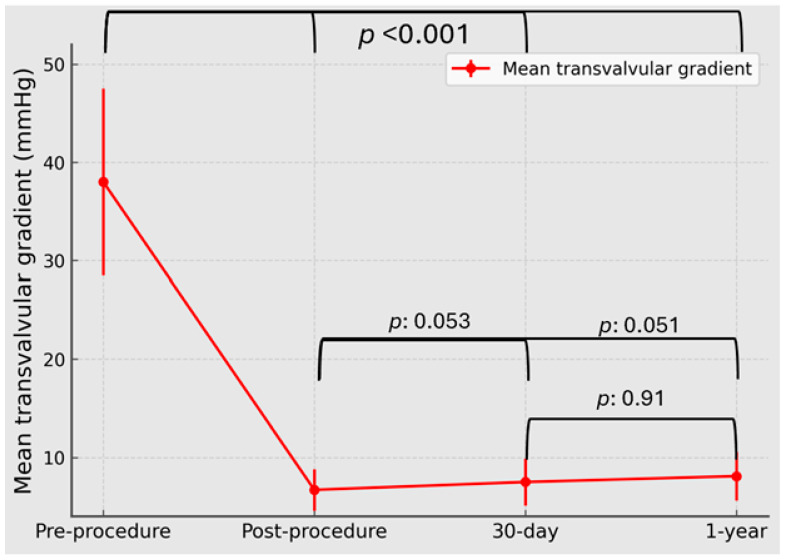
Serial mean transvalvular gradient after MyVal ViV-TAVI. Mean transvalvular gradient at baseline, post-procedure, 30 days, and 1 year after transfemoral MyVal valve-in-valve transcatheter aortic valve implantation. Values are shown as mean ± SD. Overall time effect: *p* < 0.001. Pairwise comparisons: post-procedure vs. 30 days, *p* = 0.053; post-procedure vs. 1 year, *p* = 0.051; 30 days vs. 1 year, *p* = 0.91. Observed echocardiographic sample sizes contributing data at each time point were n = 68 at baseline, n = 68 post-procedure, n = 64 at 30 days, and n = 59 at 1 year.
